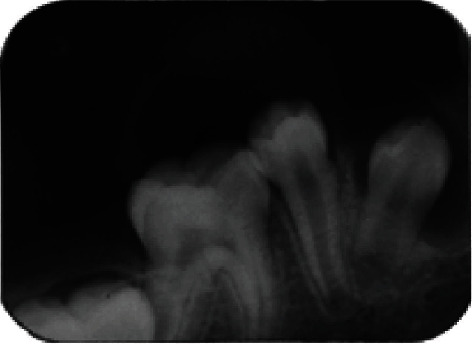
Three-month postoperative radiograph.
